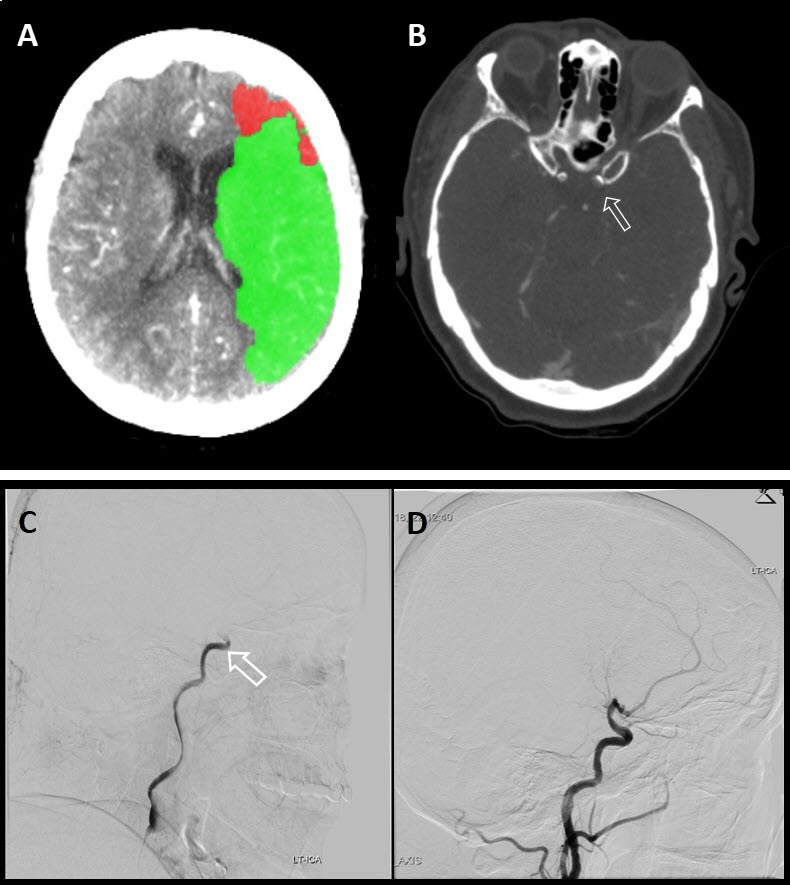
CT Brain Perfusion, CTA, Brain Angiography A: A CT perfusion algorithm calculation showing decreased perfusion in a brain area consistent with left middle cerebral artery (MCA) infarct, the green area representing penumbra. B: CT angiography showing the occluded intracranial left carotid artery with a filling defect (arrow) indicating that the artery is occluded. C: Cerebral catheter angiography showing the occluded distal left carotid artery (arrow). D: Cerebral catheter angiography after successful retrieval of the clot using a stent retriever.
